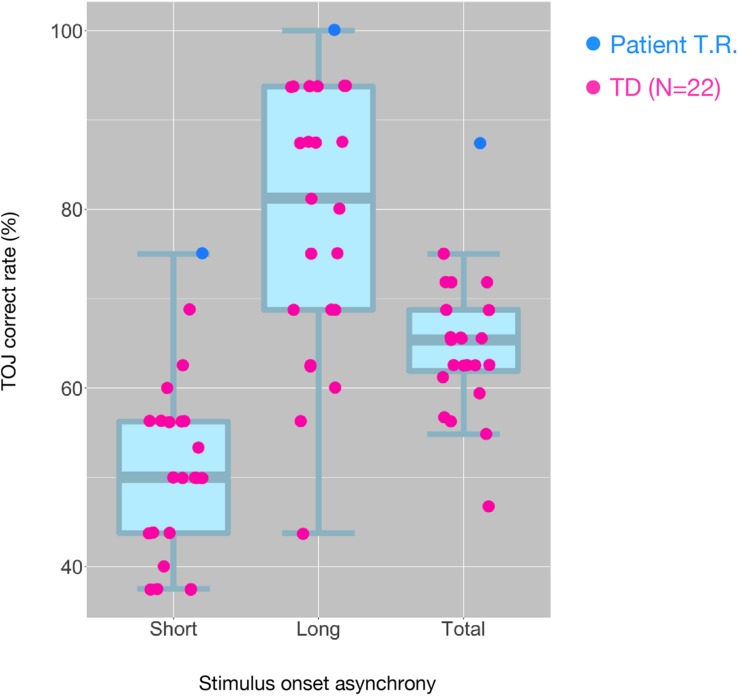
Distribution of tactile temporal order judgment (TOJ) performance of all subjects inside the MRI scanner. The box and whisker plots with the superimposed data points show the distribution of correct TOJ rates of the subjects in the “Short SOA,” “Long SOA,” and “Total (Short SOA + Long SOA)” conditions, respectively. Each box and whisker plot show the 10th, 25th, 50th, 75th, and 90th percentiles of the data. Each filled circle represents correct rate calculated from 32 trials in patient T.R. (blue) and TD controls (magenta). SOA, stimulus onset asynchrony.
